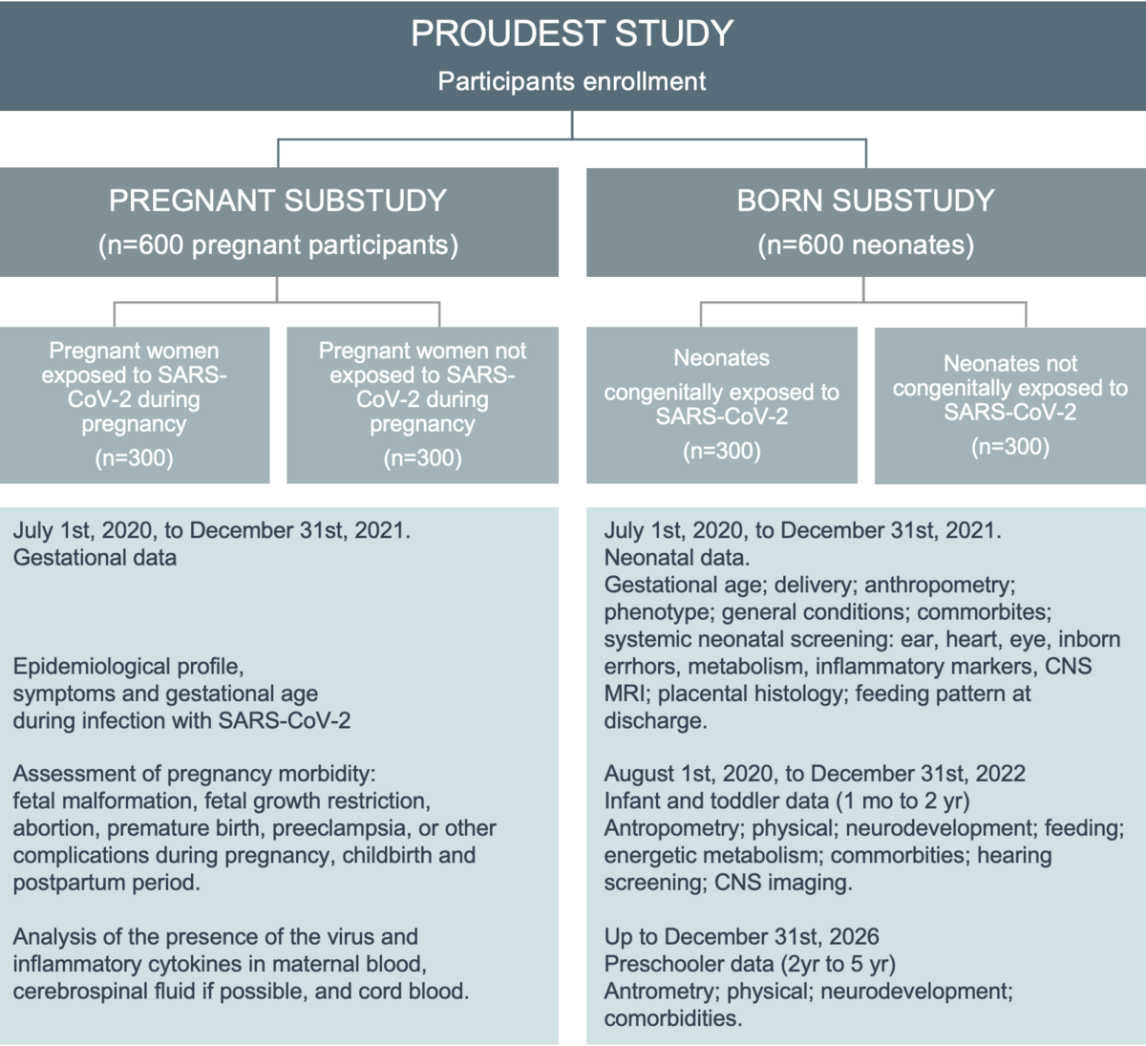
PROUDEST study design. CNS: central nervous system; MRI: magnetic resonance imaging; PROUDEST: Pregnancy Outcomes and Child Development Effects of SARS-CoV-2 Infection Study.
